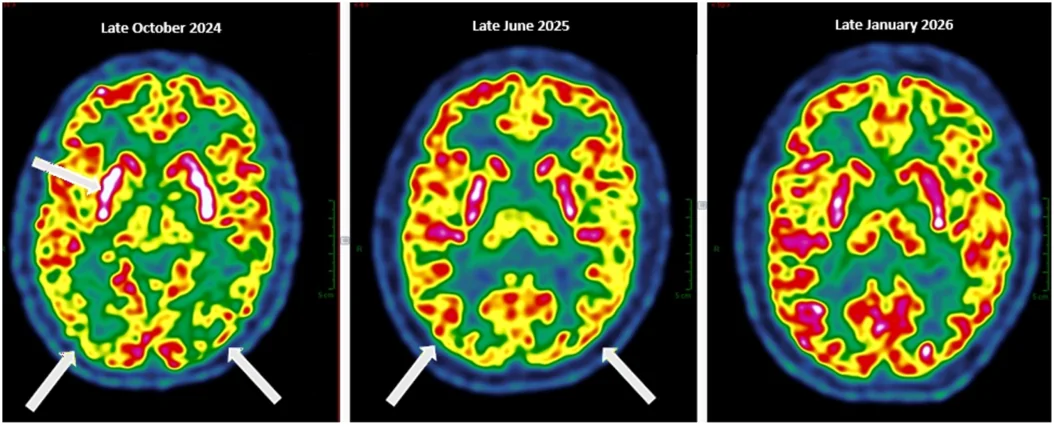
Brain fluorodeoxyglucose positron emission tomography (FDG‐PET) before and after immunomodulatory treatment. Representative axial FDG‐PET images are shown for late October 2024, late June 2025, and late January 2026. FDG‐PET examinations were performed after clinical premedication administered by the care team according to the unit's usual strategy for behaviorally constrained examinations, adapted to the patient's current treatment regimen. The initial FDG‐PET showed bilateral posterior associative cortical hypometabolism, predominantly occipital, associated with bilateral symmetric striatal hypermetabolism, without procedural sedation or documented motion artifact in the nuclear medicine report. Follow‐up FDG‐PET in late June 2025 showed marked improvement of these abnormalities. The final FDG‐PET in late January 2026 was reported as being within normal limits overall, with normalization of striatal metabolism and disappearance of the posterior associative cortical abnormalities, despite motion artifacts, reduced acquisition duration, and mild nonspecific bilateral mediofrontal hypometabolism.
